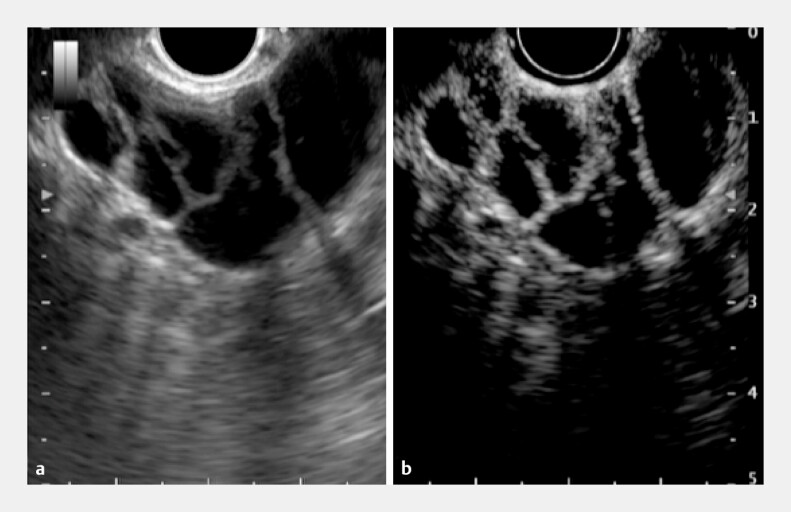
Endoscopic ultrasonography (EUS) images of a multiseptate gallbladder showing:
